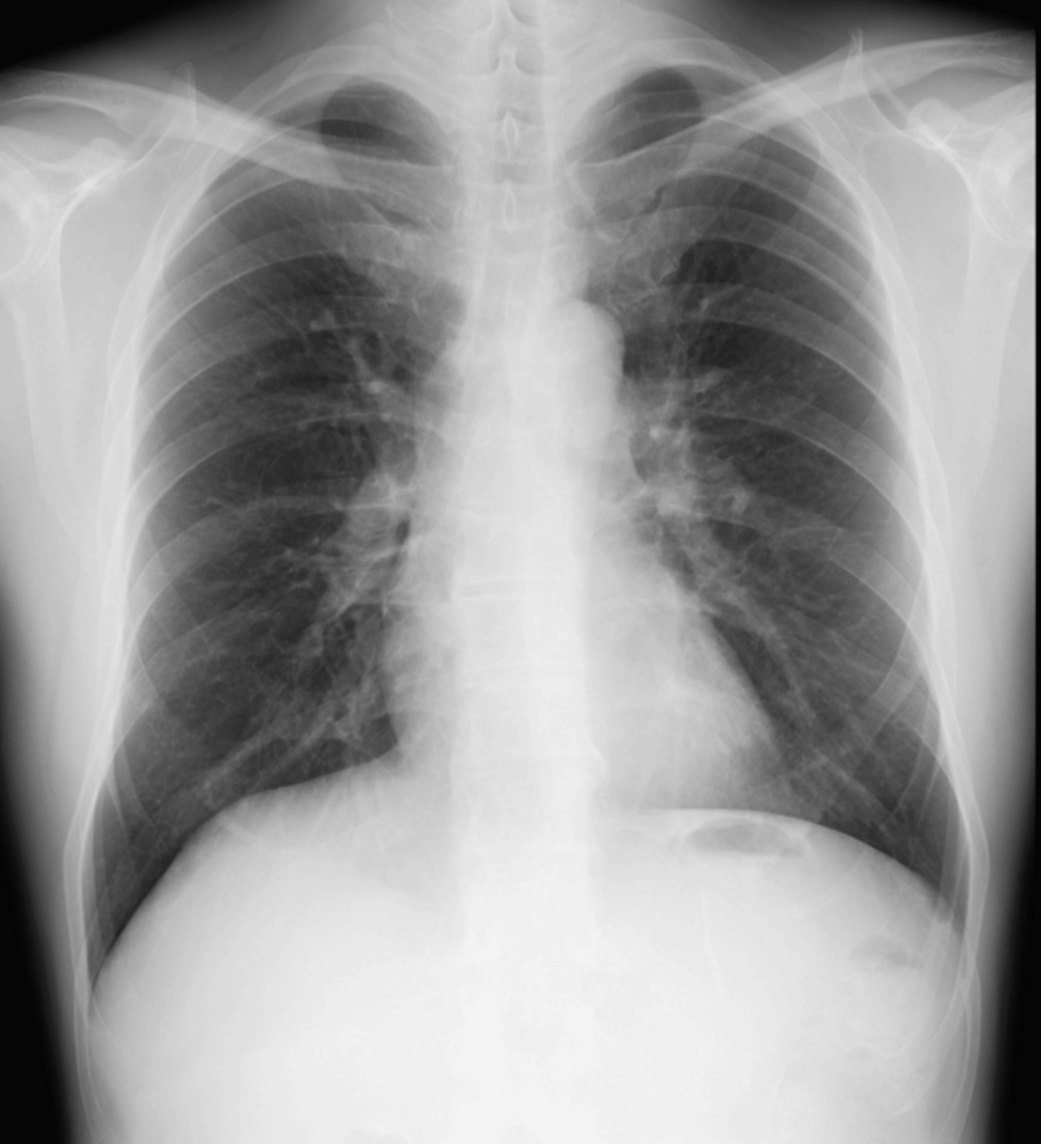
Chest X-ray. The chest X-ray revealed mild cardiomegaly.
